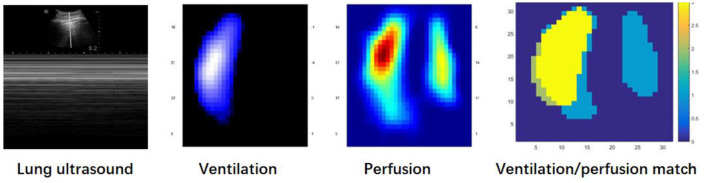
The lung ultrasound and EIT monitoring (hypoxemia occurs). 1. Lung ultrasound imaging with a convex probe (5 MHz) showed the absence of gliding sign, confirmed by the Barcode sign in M-mode at the onset of hypoxemia. 2. Functional electrical impedance tomography (EIT) images of ventilation and perfusion distribution at the onset of hypoxemia. Low-ventilated regions are marked in dark blue and high-ventilated regions in white. Regions with high perfusion are marked in red and low perfusion in blue. A massive ventilation defect was found in the left lung in which perfusion was maintained, and perfusion and ventilation matched the image at the onset of hypoxemia. Regions with high ventilation and low perfusion (indicate dead space) are marked in light green; low ventilation and high perfusion regions (indicate intrapulmonary shunt) in light blue; good ventilation-perfusion matching in yellow.
